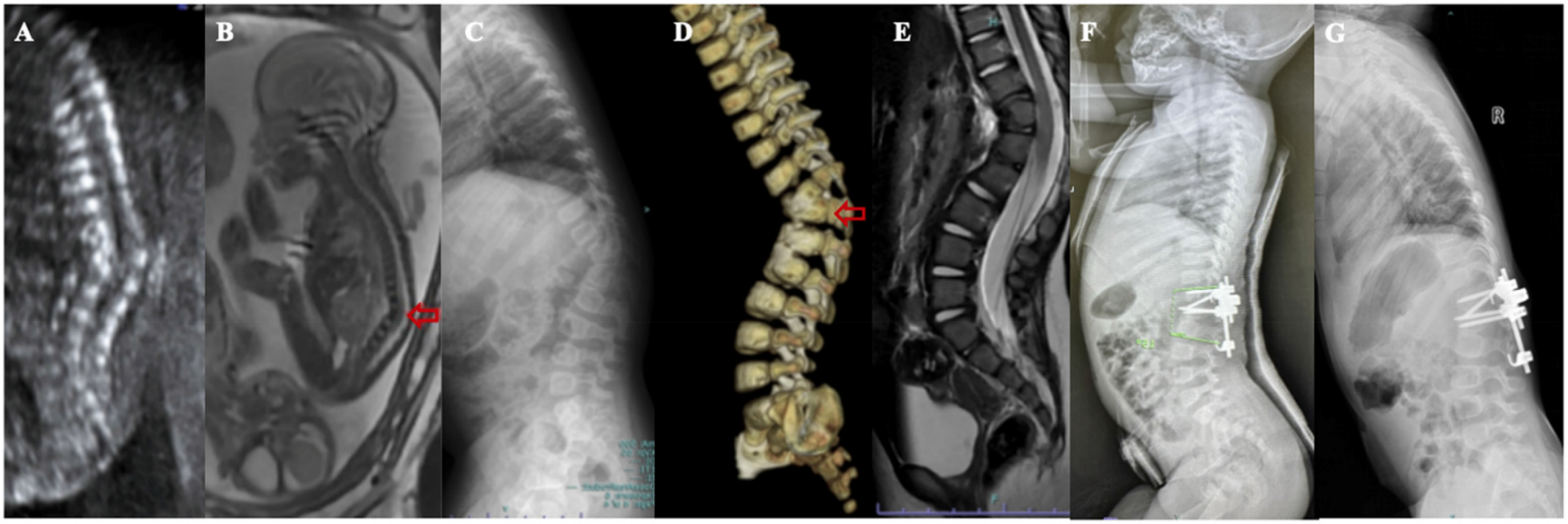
(A) A 22-week female fetus, fetal ultrasound showed obvious kyphosis deformity of the thoracolumbar segment, with localized vertebral echogenicity, disordered alignment, varying sizes, and varying widths of the vertebral interspaces; (B) fetal MRL showed congenital kyphosis in the thoracolumbar segment, with vertebral body morphology irregularities; (C–E) the girl was perfected at the age of 1 year, and the anterior bony bridge in the vertebral body of T12–L2 resulted in congenital kyphosis with a half-vertebral body to the left of the interval between T12–L1 and a 6-fractional lumbar vertebrae, SK72°; (F) schwab grade IV osteotomy was performed in L1 and grade II osteotomy in L2. Plaster external fixation was performed one after surgery, SK15°, kyphotic correction rate of 79.2%; (G) Reexamination 37 months after surgery showed SK16° and almost no loss of correction rate.
